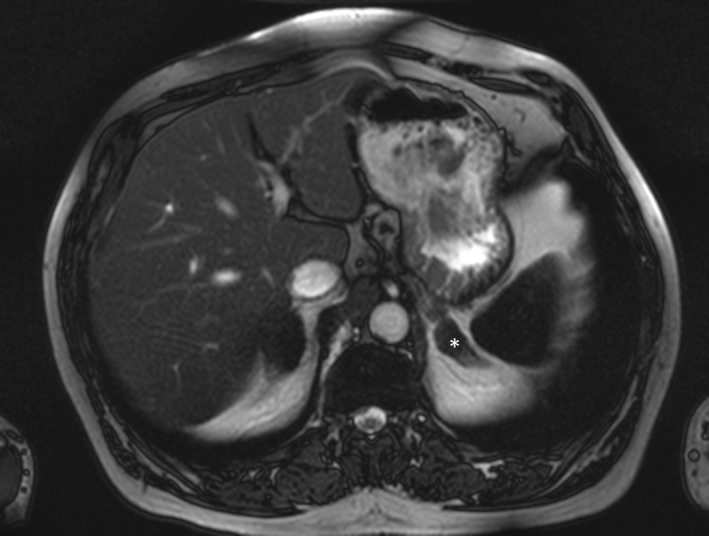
MRI father. Abdominal MRI of the patient's father revealed a nodule in the left adrenal gland (“_*_”).
